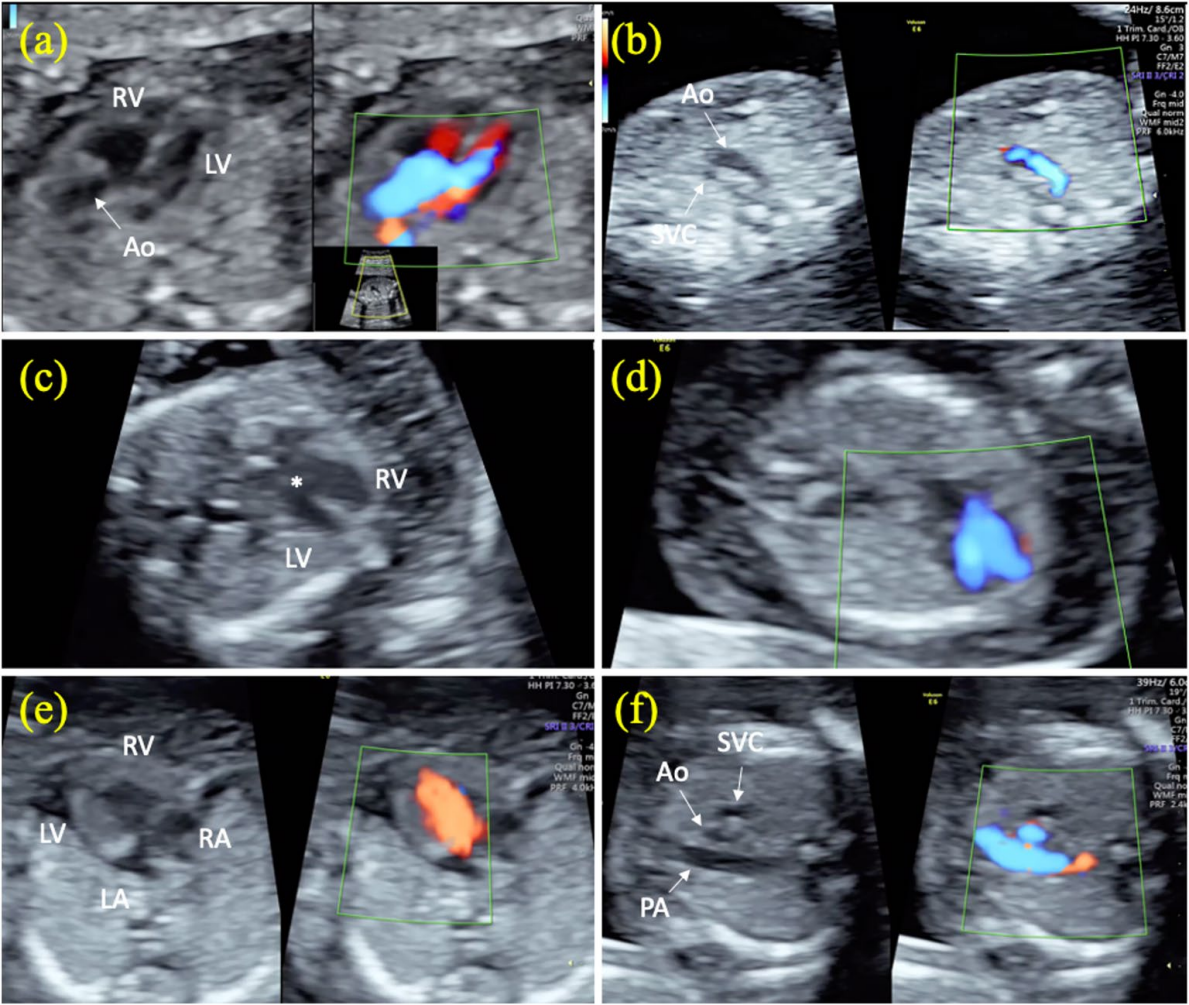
First trimester fetal echocardiographic images of tetralogy of Fallot, atrioventricular septal defect, and hypoplastic left heart syndrome. Tetralogy of Fallot (a, b). Note the course of the aorta in the left ventricular outflow tract (overriding aorta) and color Doppler image in the same section (a). There are two vessels (aorta and superior vena cava) in the 3‐vessel view of the same fetus (b). Atrioventricular septal defect (c, d). Asterisk indicates endocardial cushion defect in gray scale (c) and ventricular filling on color Doppler (d). Hypoplastic left heart syndrome (e, f). Appearance of left ventricular hypoplasia in gray scale and color Doppler (e). Note that the aorta is hypoplastic in the 3‐vessel view in the same fetus (f). Ao, aorta; LA, left atrium; LV, left ventricle; PA, pulmonary artery; RA, right atrium; RV, right ventricle; SVC, superior vena cava.
